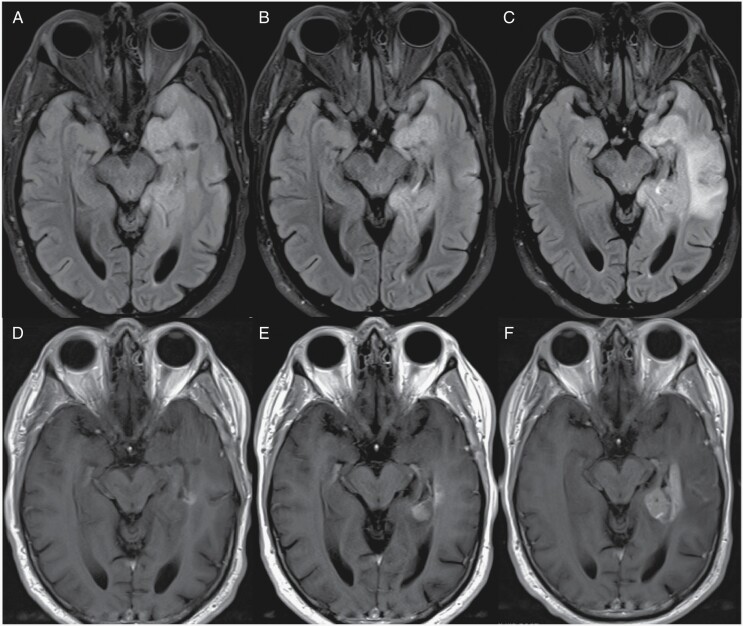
BT-RADS criteria used for response assessment of a WHO Grade 2, IDH wild-type astrocytoma. Axial FLAIR and T1 postcontrast images at 7 months (A, D), 10 months (B, E), and 13 months (C, F) after surgical resection are shown. At 10 months, a score of 3c (recommendation for decreased interval of follow-up) was assigned given worsening enhancement (E) and marginally increased FLAIR hyperintensity (B). At 13 months, a score of 4 (recommendation to change clinical management) was assigned given further worsening of both enhancement (F) and FLAIR hyperintensity (C) over multiple studies.
